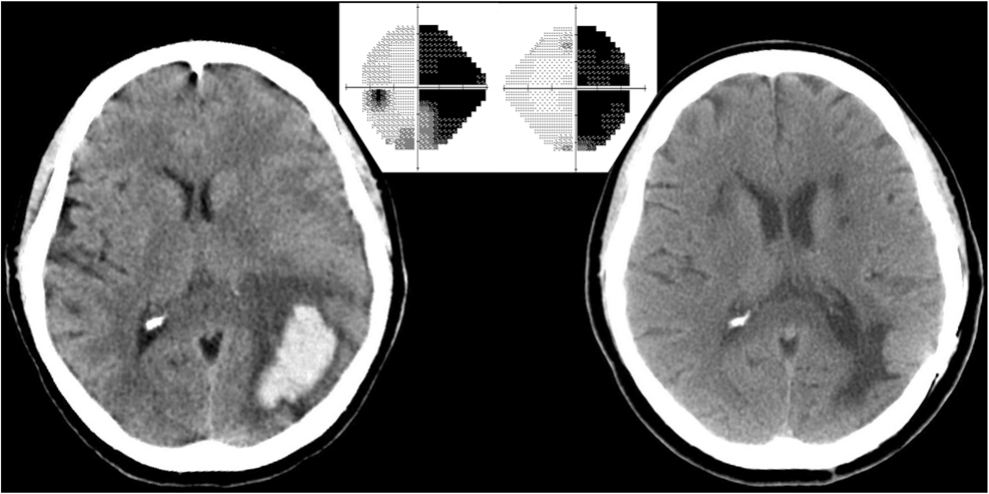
CT scan showing an acute left parietal hematoma, causing a right homonymous hemianopia. A CT scan performed 5 months later shows damage to the left optic radiations. The visual field cut never recovered
